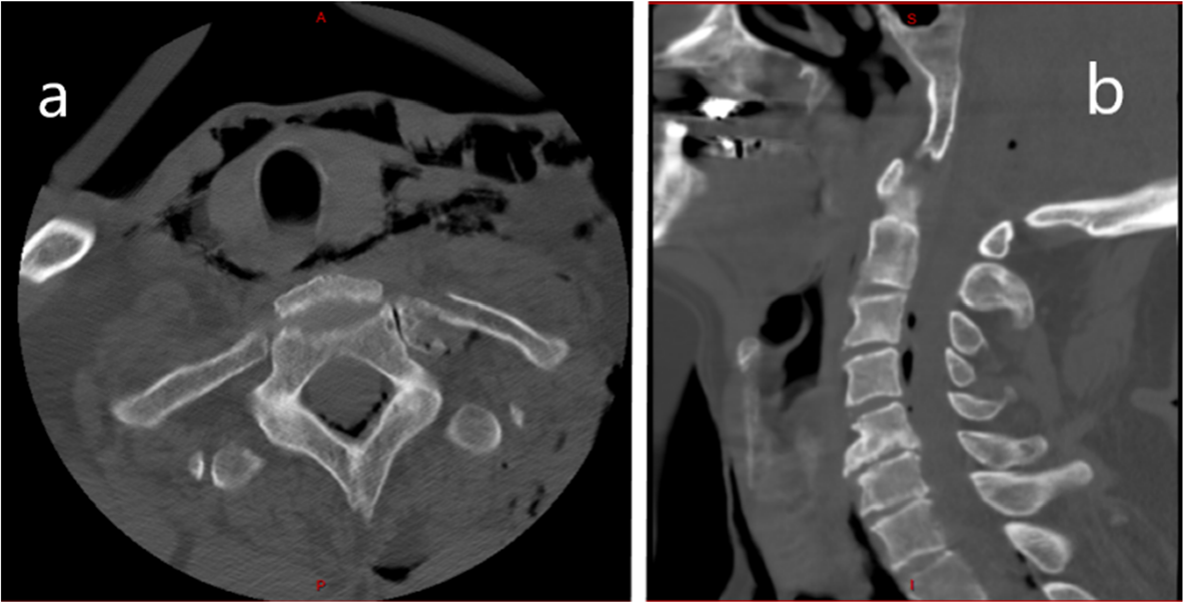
Initial cervical spine computed tomography, axial (a) and sagittal (b) view, showing pneumorachis on multiple levels
